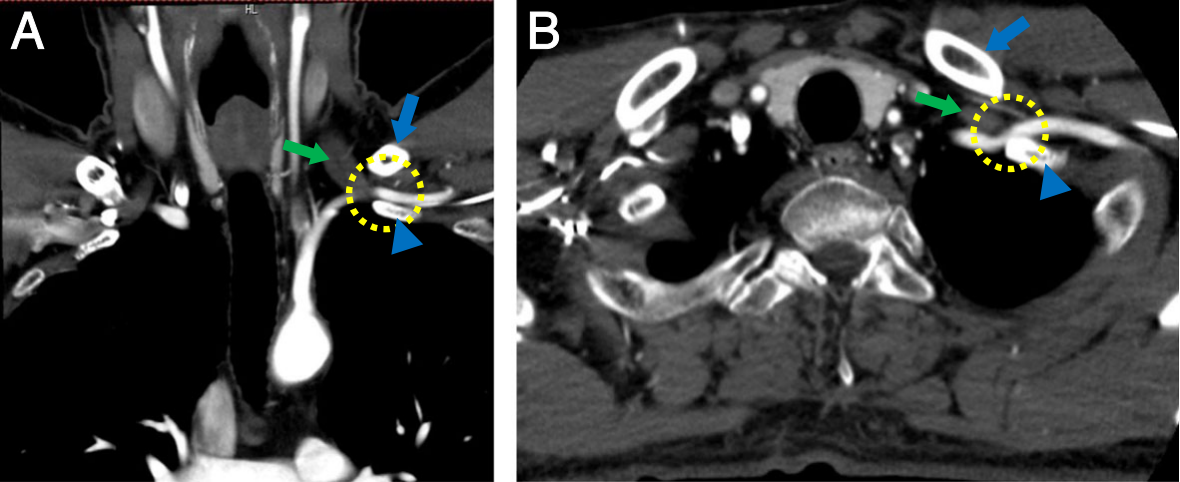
Preoperative enhanced CT (a coronal view, b axial view). Yellow dotted circles indicate the slightly compressed left subclavian artery in the left costoclavicular space. (blue arrow: clavicle, blue triangle: the first rib, green arrow: anterior scalene muscle)
